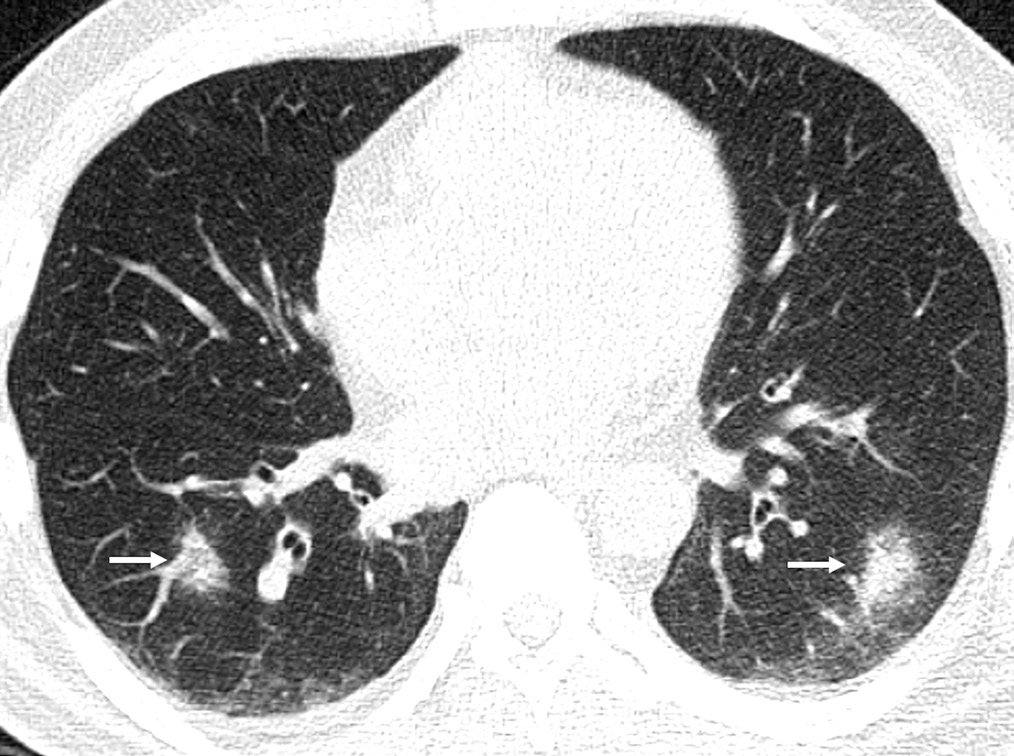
47-year-old male with COVID-19 infection. Axial CT image showing typical and most common imaging manifestation – bilateral, peripheral, and round ground-glass opacities (white arrows).
